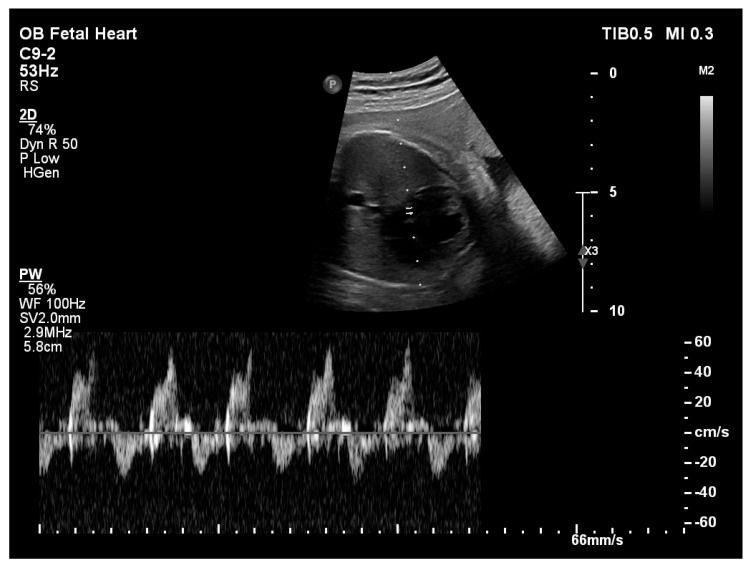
Spectral Doppler assessment of the mitral valve on fetal echocardiography showing a biphasic transmitral inflow pattern.
